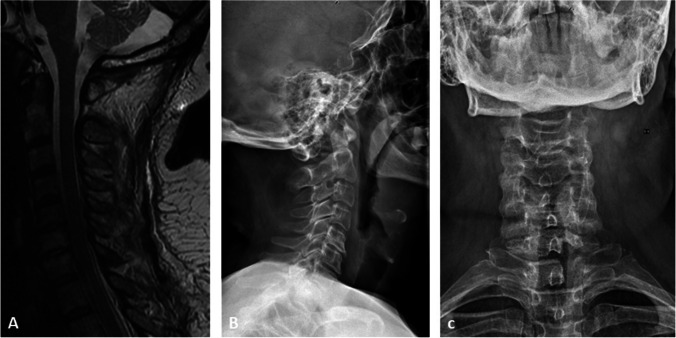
A sagittal MRI T2 sequence (a) and a lateral (b) and anteroposterior (c) cervical radiography performed at 3 and 6 months after surgery, respectively, showed a preservation of the spinal alignment
